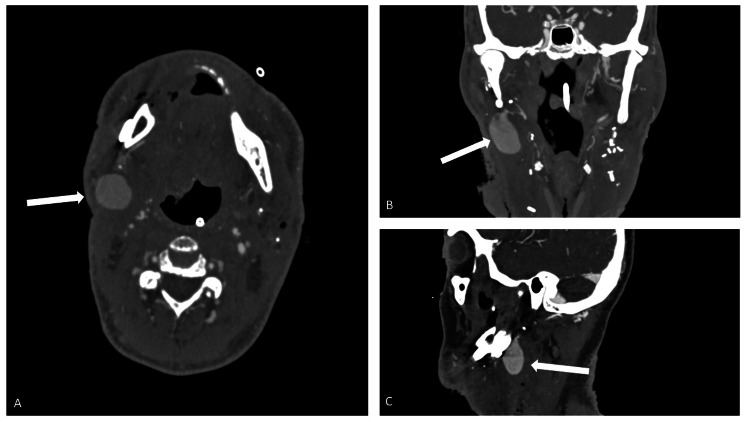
Axial (A), coronal (B), and sagittal (C) images of a neck CT angiogram demonstrating a 2.9cm pseudoaneurysm from the external maxillary artery. The pseudoaneurysm sac extends inferolaterally into the right neck post-surgical bed.
